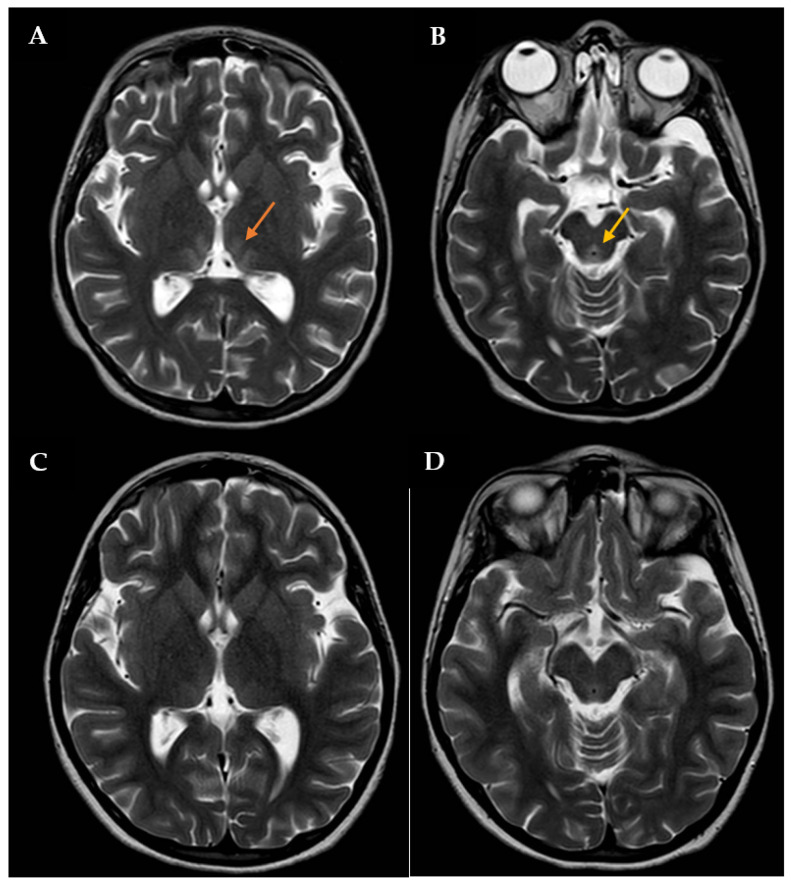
Brain MRI with contrast showing T2 signal hyperintensity involving the medial thalami (orange arrow in A) and the periaqueductal region (yellow arrow in B). The patient was initially treated with high-dose intravenous thiamine, then with oral thiamine on a daily basis. A new brain MRI showing a significant improvement in lesions was assessed one month after disease onset (C,D).
